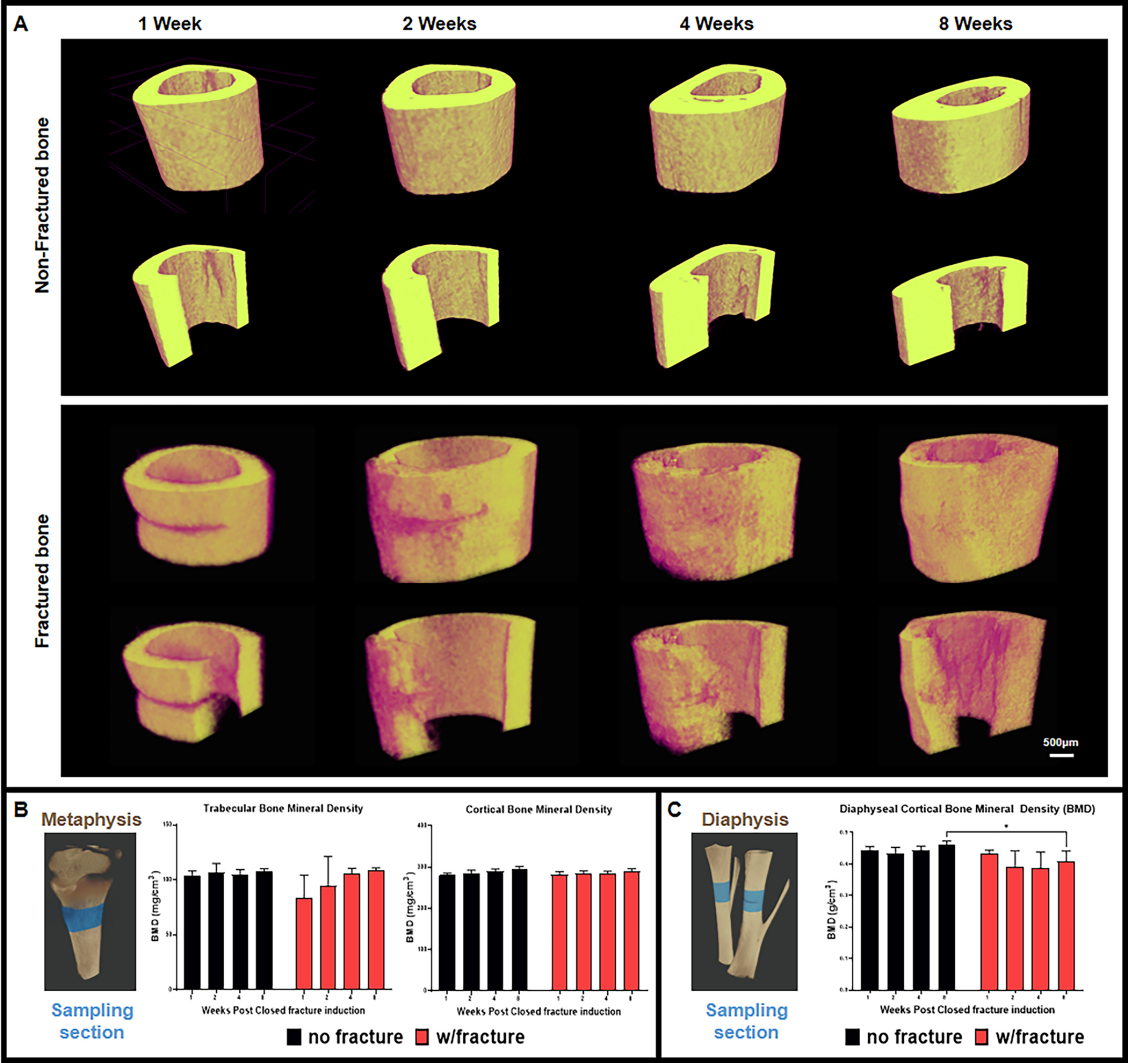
Whole and sectioned 3D reconstruction of Micro‐CT data of intact tibia bone tissue and fracture site sampled at 1 week, 2 weeks, 4 weeks, and 8 weeks (A). Bone mineral density (BMD) measurements comparing trabecular and cortical bone density along the metaphyseal area and (B) comparison of cortical BMD at the diaphyseal segment of the non‐fractured and fractured tibia bone tissue sampled at 1, 2, 4, and 8 weeks post‐fracture induction (Scale bar = 500 µm).
